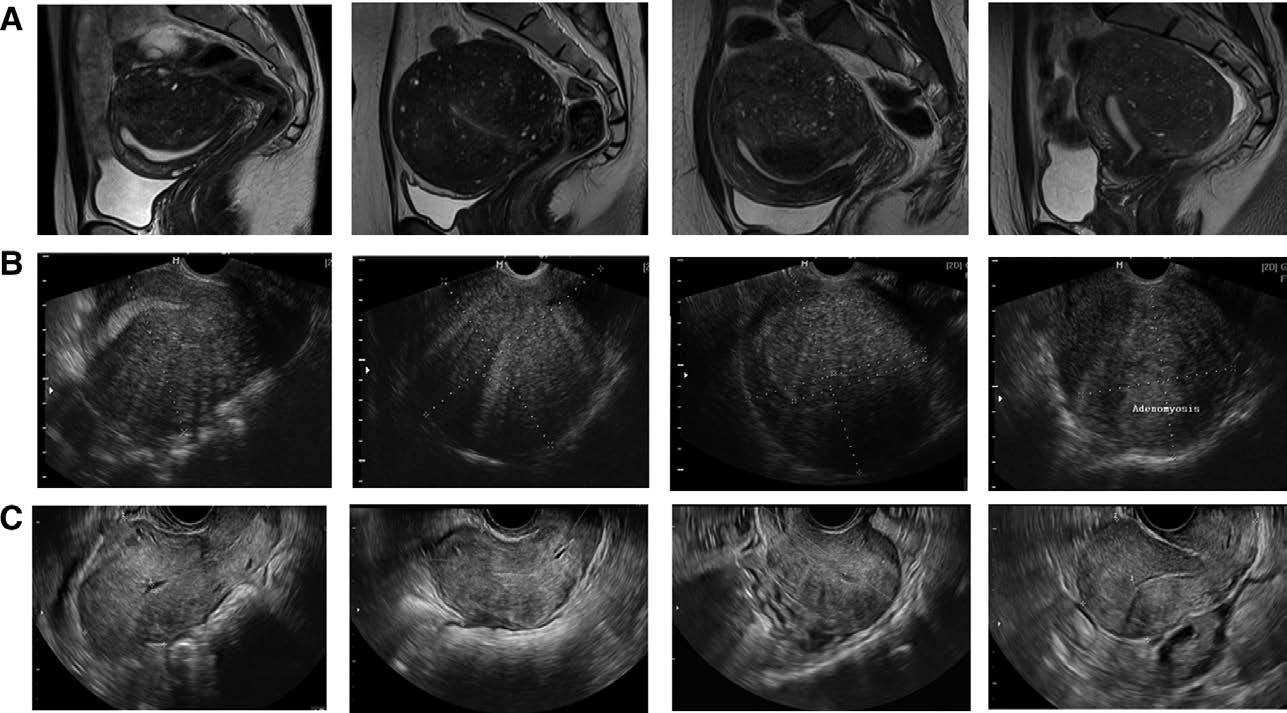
Representative images of MRI and trans-vaginal ultrasonography. (a) MRI scans of 4 different patients show adenomyosis. (b) Trans-vaginal ultrasonography was used to measure the adenomyotic lesion. (c) Trans-vaginal ultrasonography 3 to 6 mo follow-up after surgery. MRI = magnetic resonance imaging.
